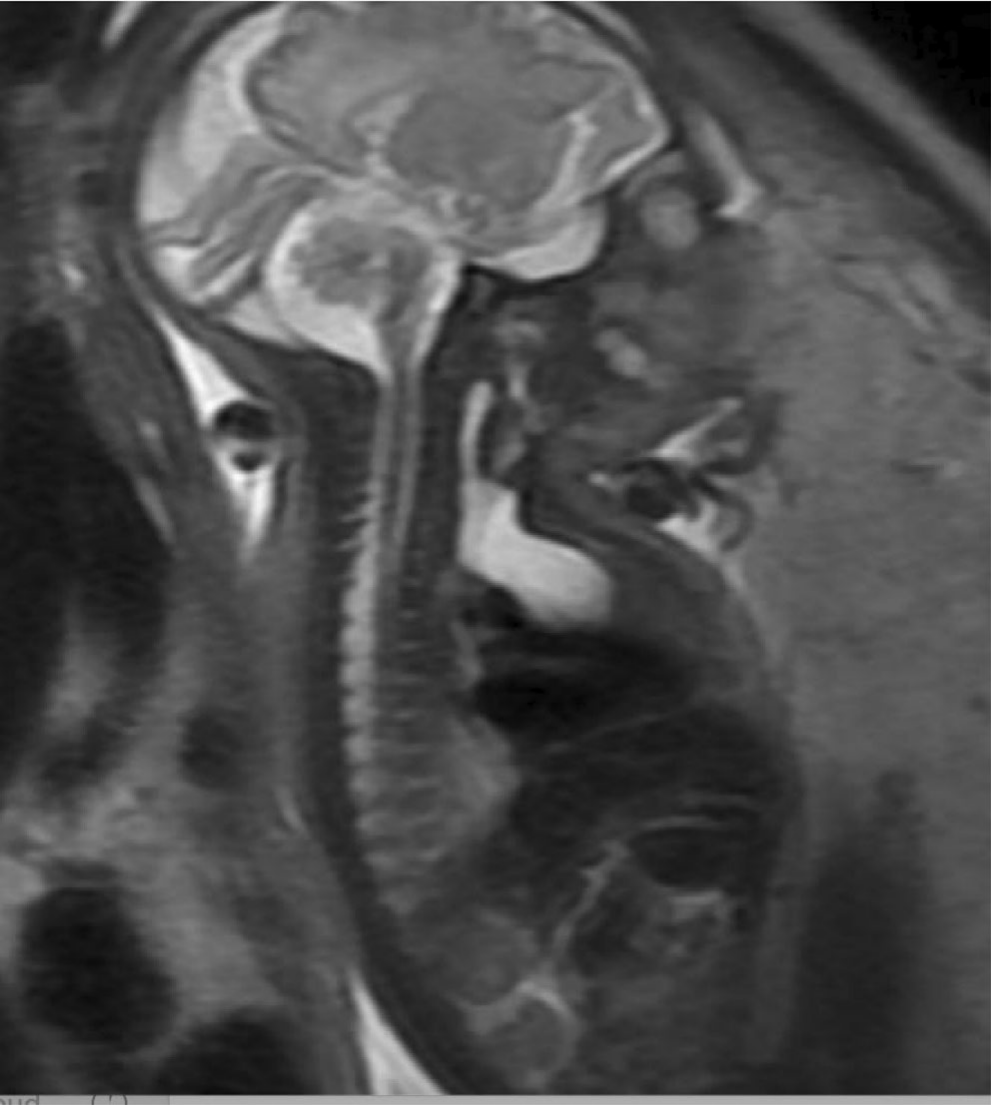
Esophageal duplication cyst at 31 weeks 6 days of pregnancy. Magnetic resonance imaging image showing a fetal cystic mediastinal mass, tangent to the oesophageal wall and communicating with the oesophageal lumen over a distance of 18 mm.
